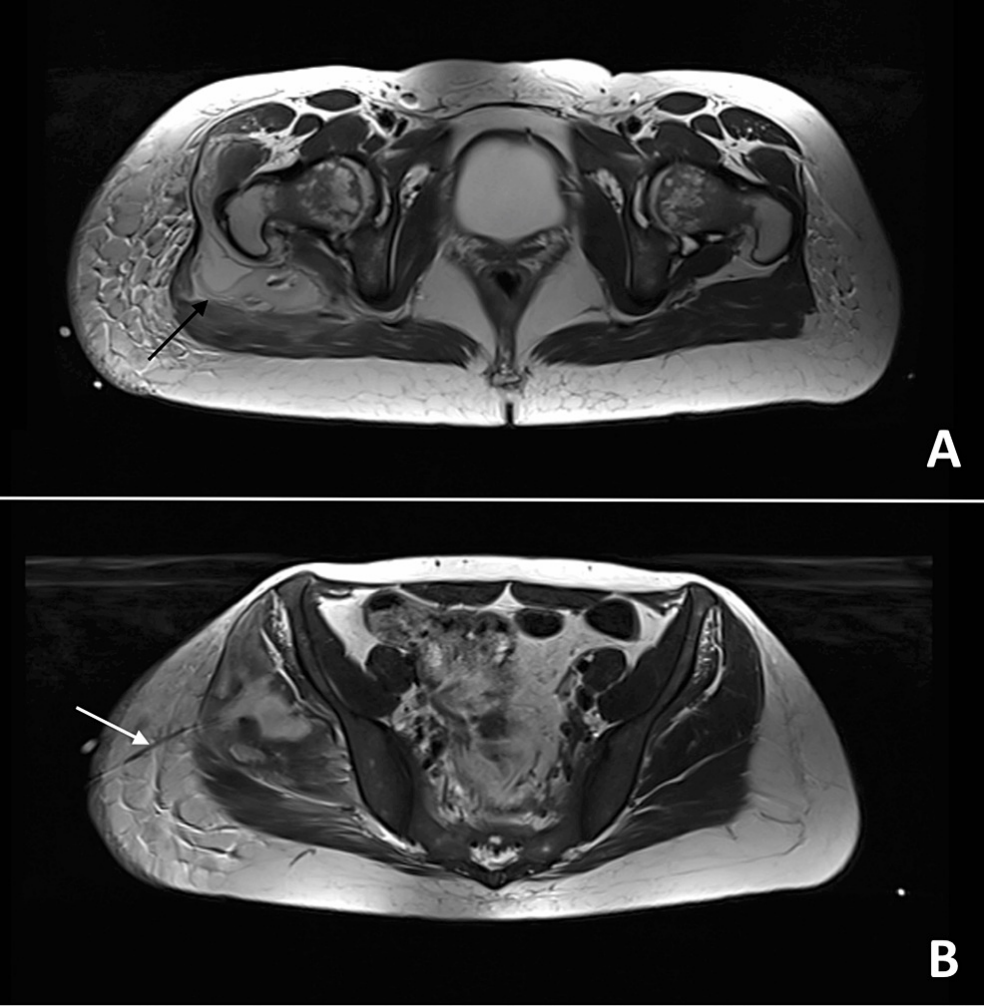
Pelvic and upper right leg MRI scan (T2): transverse plane (image A) and coronal plane (image B). In image A, we can confirm the bigger intramuscular collection (black arrow) in the right gluteal with higher resolution (compared to a CT scan) of adjacent fat densification. In image B, it was also possible to check the correct placement of the drain (white arrow). In this same MRI (in more distal sections), it was also possible to discover a new isolated thick-walled liquid collection, measuring 13 mm in the muscle belly of the rectus femoris (proximal third of the thigh), suggestive of an abscess.
